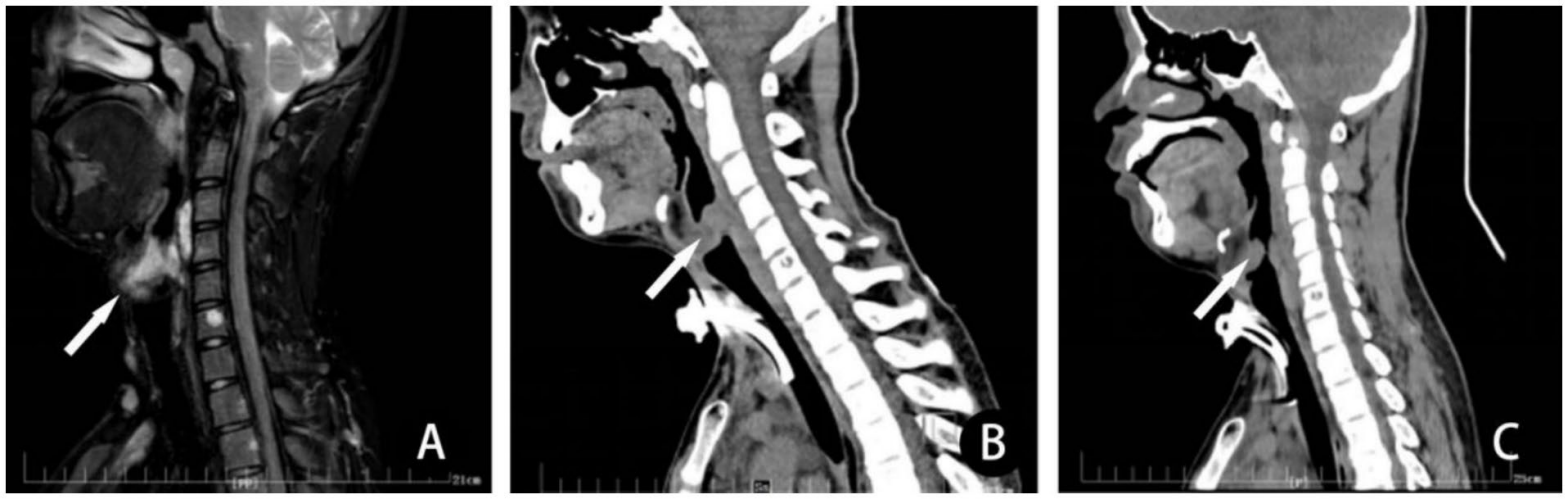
In a patient with LM involving the pharynx, (A) preoperative T2-MRI sagittal scan, revealed that the lesion involves and obstructs the airway (arrow) significantly. Three depressions sign was obvious when he walked fast. (B) He received a session of sclerotherapy with combination of ethanol and bleomycin after tracheotomy. Computerized tomography scan 1 month after treatment revealed severe obstruction of airway due to post-sclerotherapeutic reactive edema. (C) Computerized tomography scan 6 months after sclerotherapy demonstrated airway obstruction improved. Then, the tracheotomy tube was removed. There is a decrease in the size of the mass of the LM in the posterior subglottic LM lesion and little change in size of the mass in the anterior subglottic LM lesion. Despite this residual narrowing, the patient suffered no further sign or symptoms of airway obstruction.
